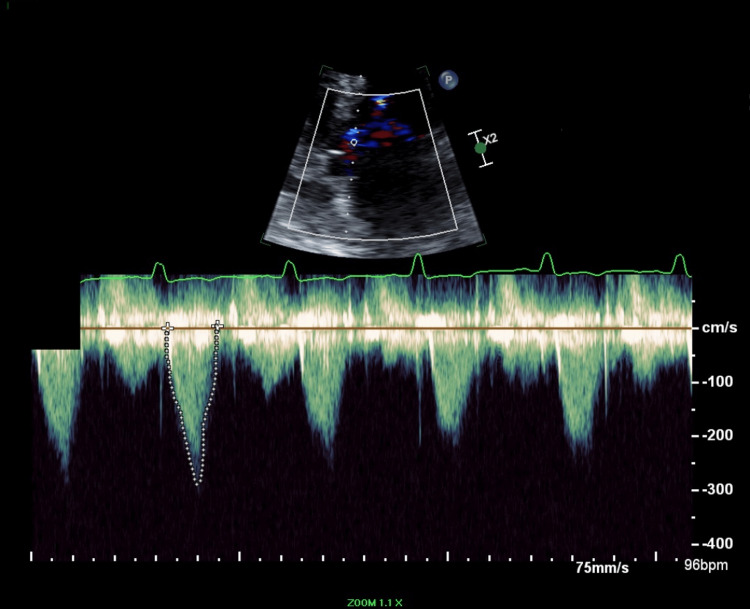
Transthoracic echocardiography image Late peaking continuous wave Doppler for left ventricular outflow tract, which suggests dynamic obstruction with evidence of chordal systolic anterior motion of the mitral valve.
